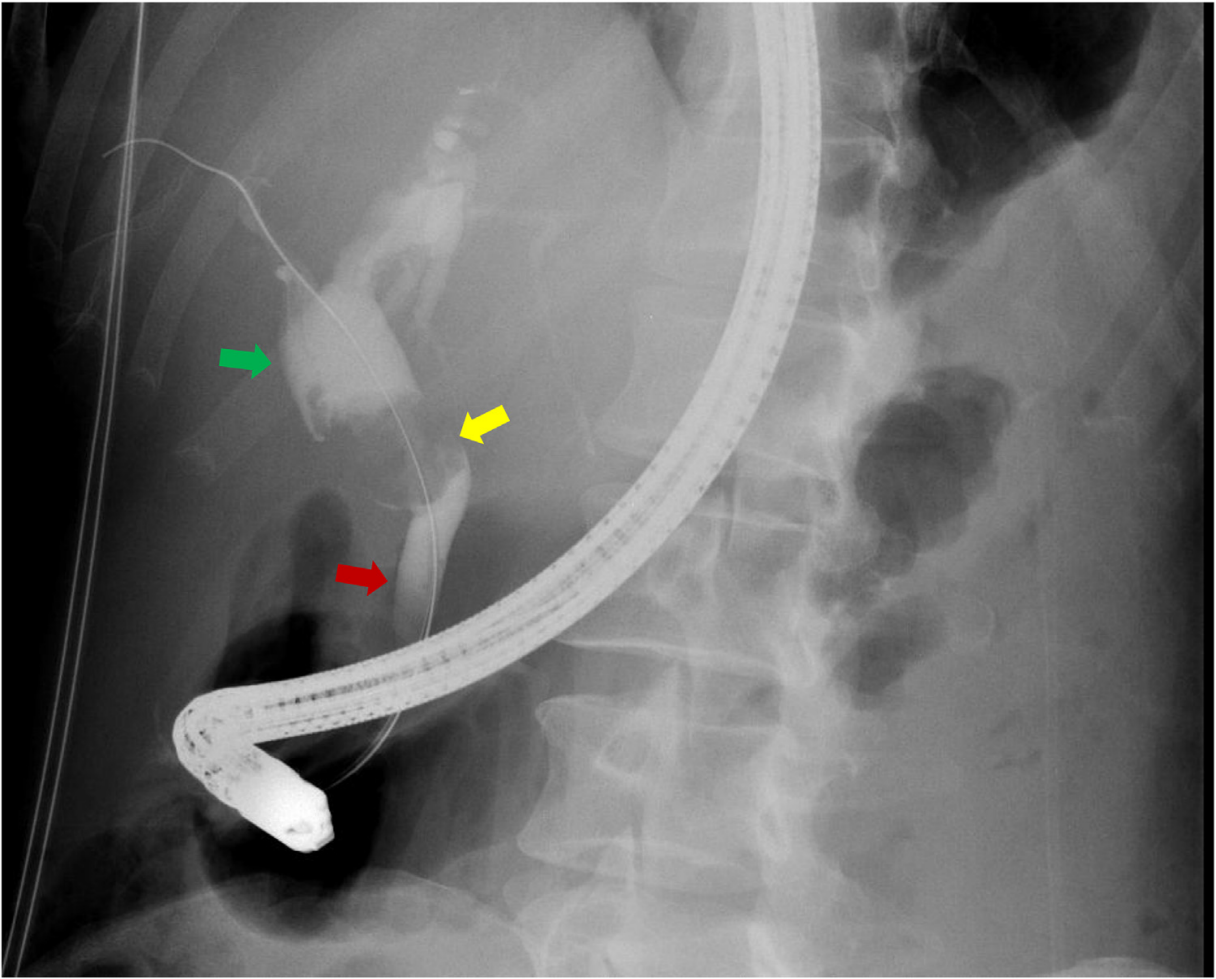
Endoscopic retrograde cholangiopancreatography (ERCP): Radiographic image from ERCP procedure demonstrates superior common hepatic duct dilatation (green arrow) and mass-like filling defect and narrowing produced by the gallbladder at the level of the expected confluence of the common hepatic duct, cystic duct, and common bile duct (yellow arrow), with the common bile duct opacified further inferiorly (red arrow).
